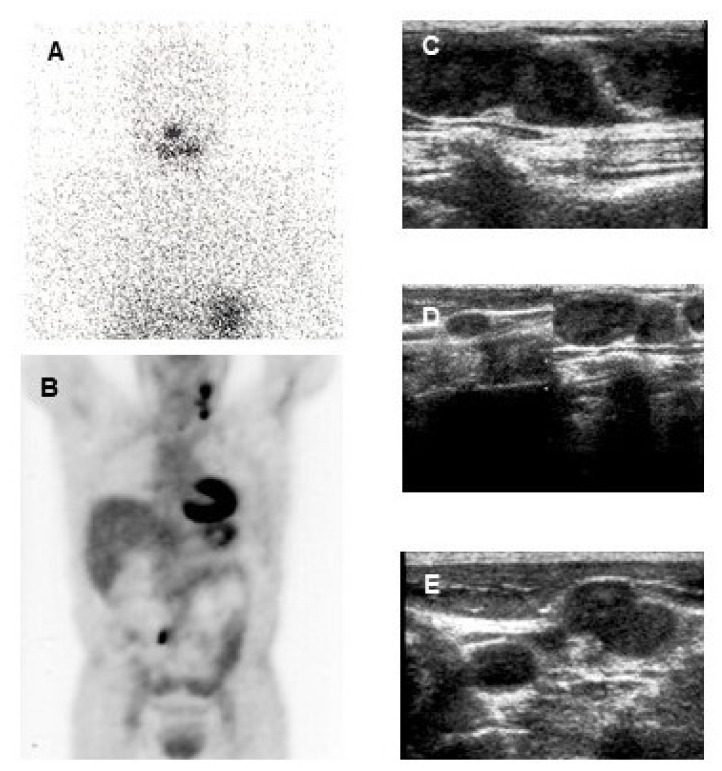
37-year-old woman with a papillary thyroid cancer (tall cell variant) with a post-operative TSH-stimulated serum thyroglobulin (Tg) level of 230 ng/mL. Post-therapy ^131^I whole-body scan (A) did not show any area of increased uptake. ^18^F-FDG PET-CT (B) evidenced multiple areas of increased uptake in the left latero-cervical region and homogeneous cardiac ^18^F-FDG uptake (that is not an infrequent finding, also in patients without overt cardiac disease). Ultrasound (C,D,E) evidenced multiple suspicious lymph-nodes in the left latero-cervical region, with round shape, loss of echogenic hilus and micro lobulated margins. At fine needle aspiration cytology confirmed lymph node metastases from the papillary thyroid cancer and the Tg concentration was elevated (3800 ng/mL) in the aspirate fluid.
